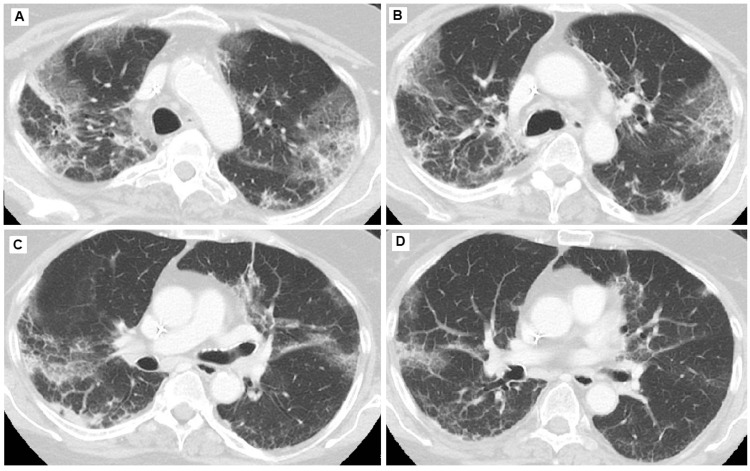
(A-D): CT scan of the chest on day 10 of admission showing interval increase in interstitial findings. CT: computed tomography
